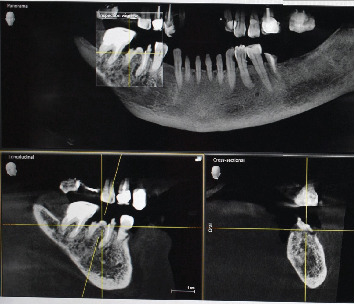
CBCT for treatment planning.
